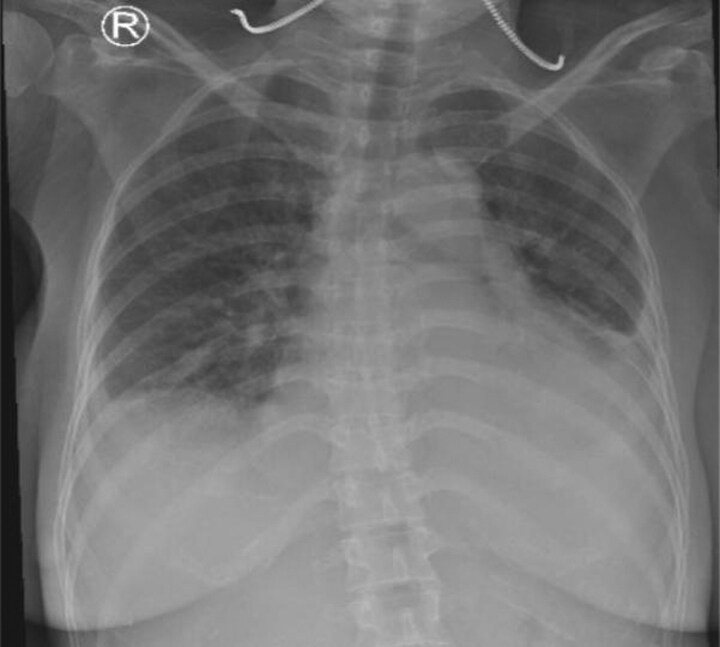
Chest X-ray demonstrated cardiomegaly, bilateral pleural effusion, and pulmonary vascular cephalization.
